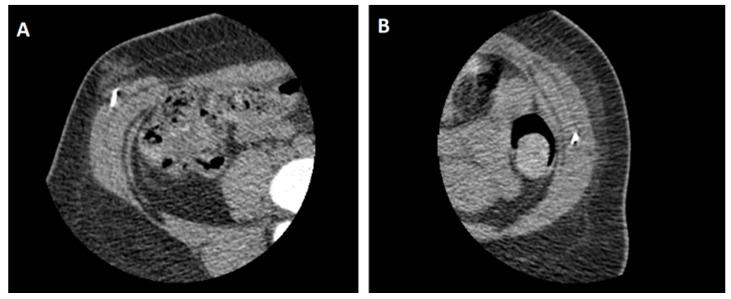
Still images of botulinum toxin A (BTA) instilled under CT-fluoroscopic guidance. The radiodense needle tip can be seen infiltrating into the right external oblique muscle (A) and the left internal oblique muscle (B).
